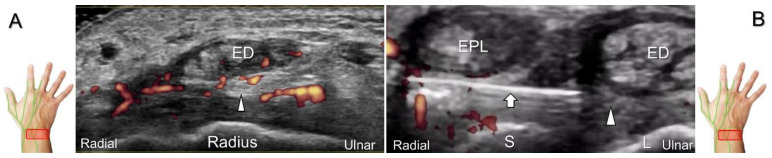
Sonographic imaging (short-axis view) is performed to assess the posterior interosseus nerve entrapment due to synovitis in rheumatoid arthritis (A). Ultrasound-guided injection (B). Arrowheads: posterior interosseus nerve; arrow: needle; EPL: extensor pollicis longus tendon; ED: extensor digitorum communis tendon; S: scaphoid; L: lunate.
